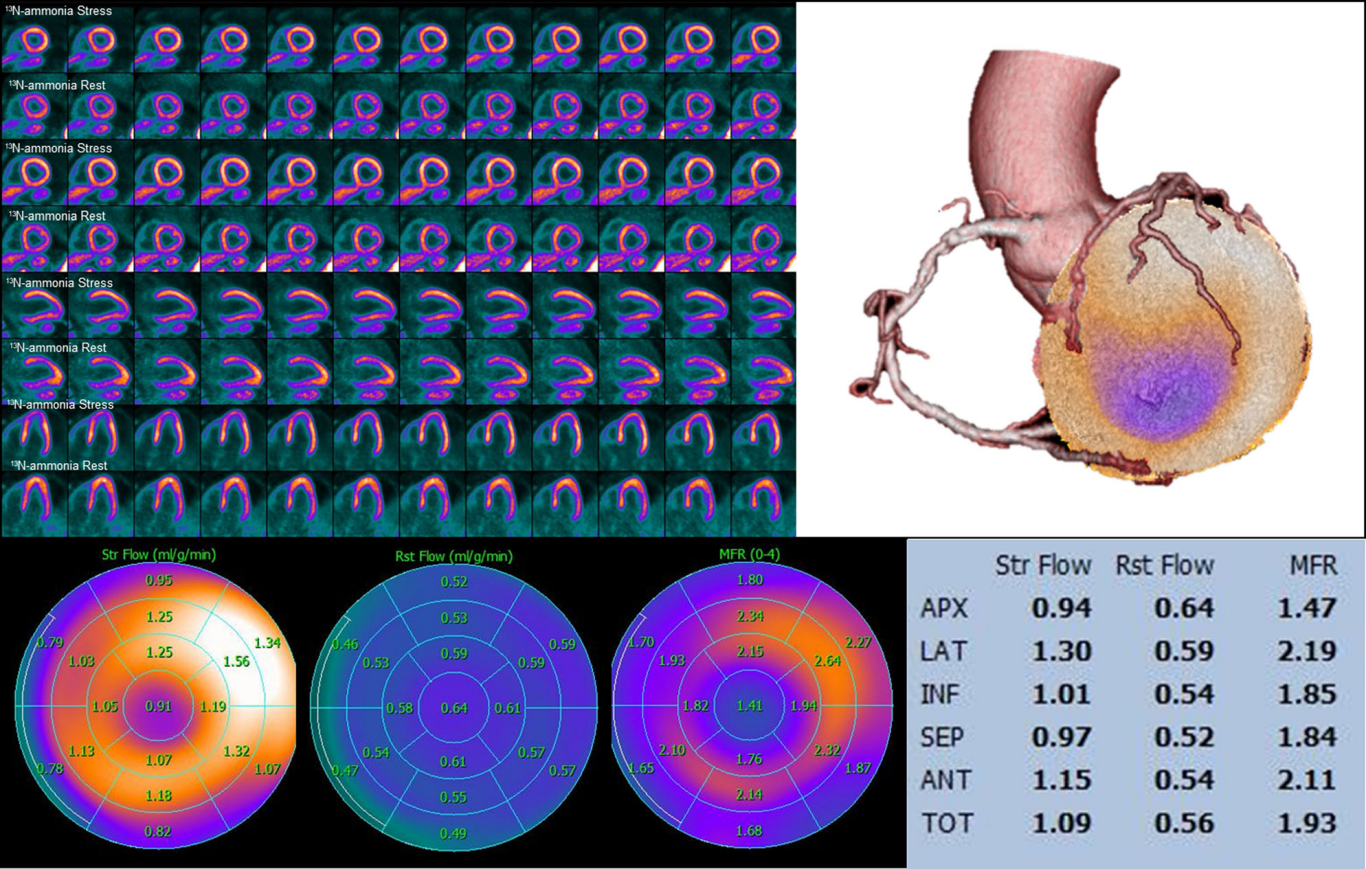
CMD in a 66 years old patient with apical HCM. Left upper Panel shows ^13^N-ammonia PET perfusion study (short axis, horizontal and vertical long axis slices) with evidence of subendocardial perfusion defect in stress which did not correspond to a coronary artery supply territory as assessed in fused coronary computed tomography angiography and ^13^N-ammonia PET images (right upper panel). Lower panel shows quantitative PET analysis with evidence of CMD depicted by globally pathological MFR (Total MFR 1.93). The apical segments show markedly reduced hyperemic MBF and MFR while the microcirculature is also compromised on the non-hypertrophic inferior and septal LV-segments
